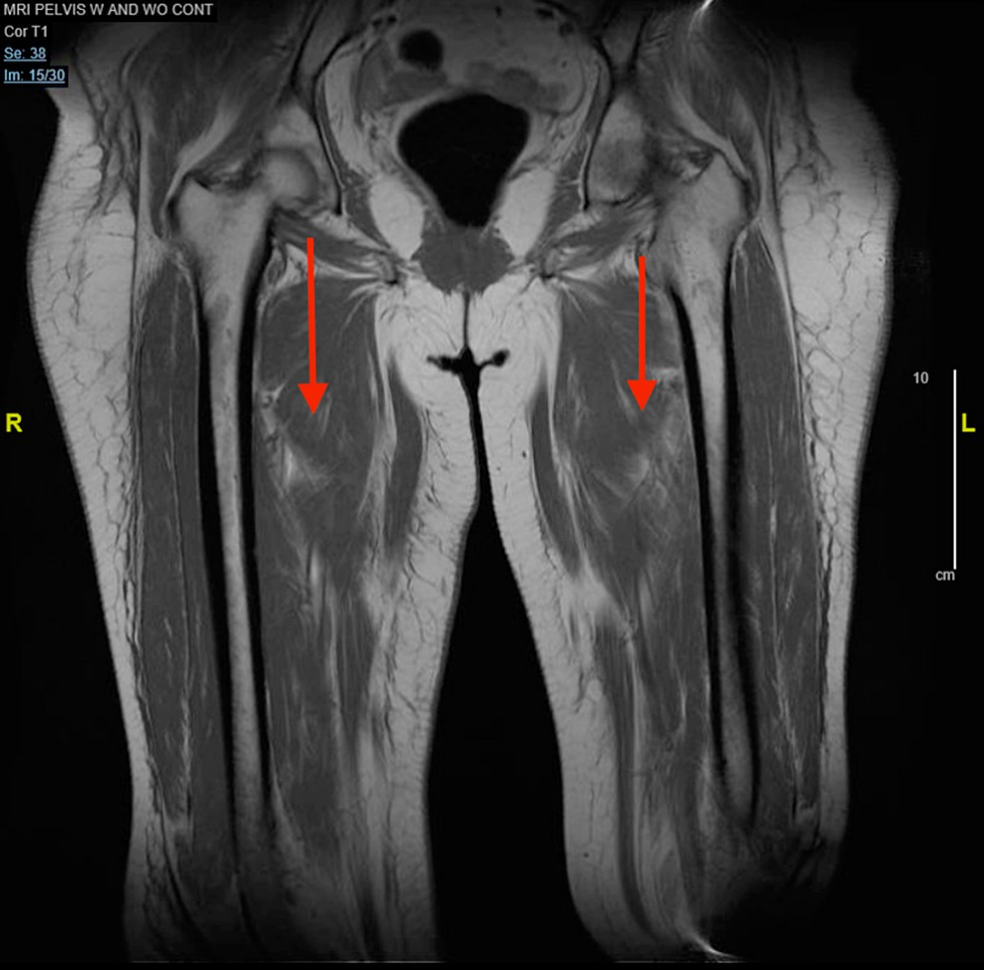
Coronal MRI of pelvis Arrows demonstrate muscular edema in the thighs bilaterally.
